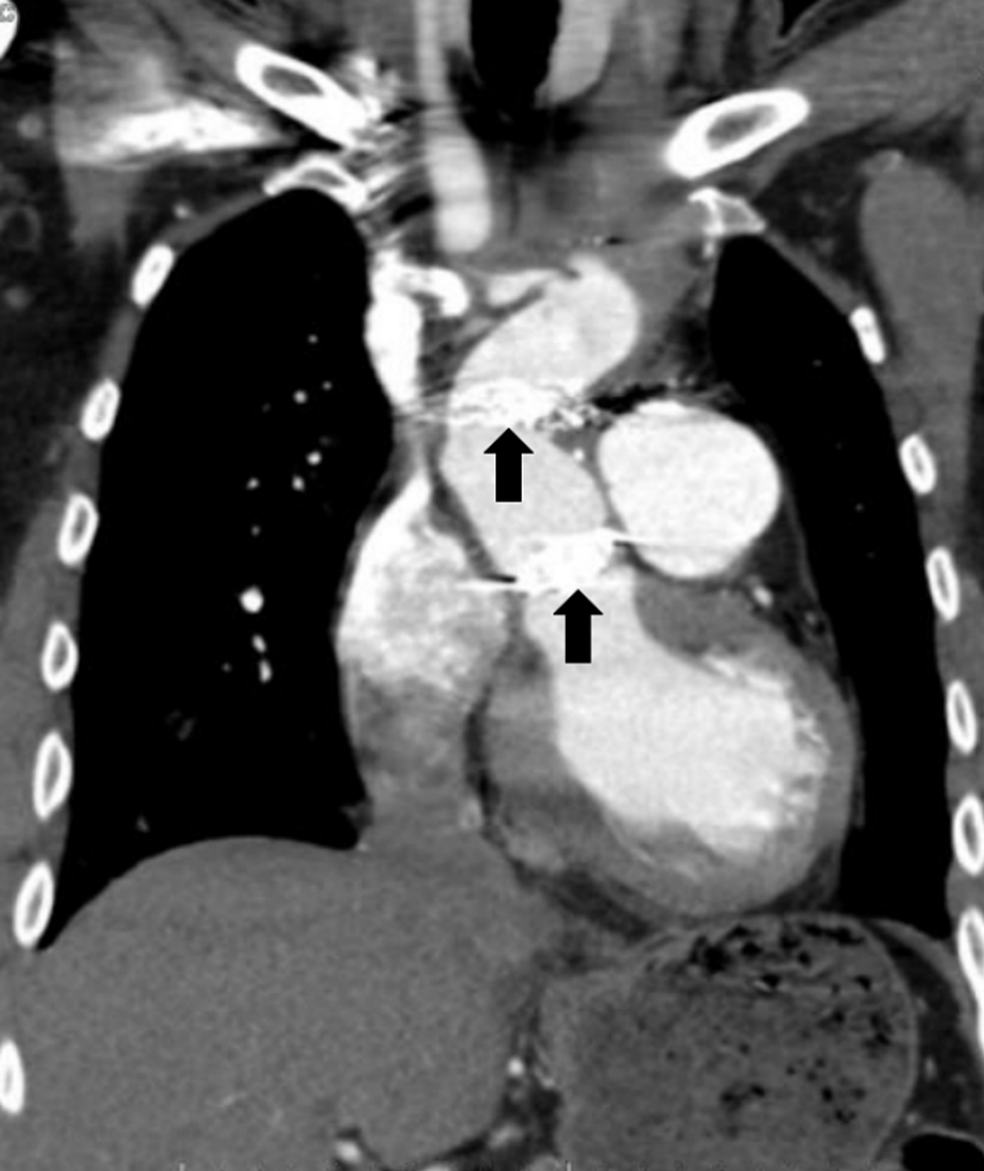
CT angiogram of the chest (coronal view) The prior aortic repair anastomotic sites can be easily visualized in this plane (indicated by arrows).
